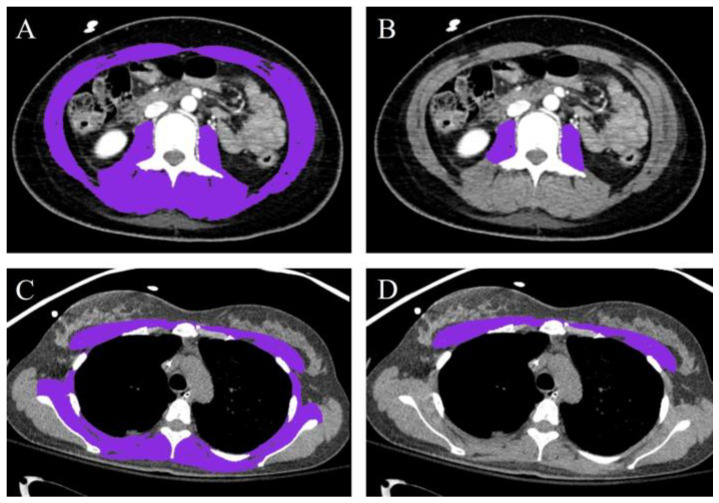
Cross-sectional CT images of the SMA indicated in purple. (A) L3 level SMA, (B) psoas muscle area, (C) T4 level SMA, and (D) pectoralis muscle area. Abbreviations: CT, computed tomography; L3, the third lumbar vertebra; SMA, skeletal muscle area; T4, the fourth thoracic vertebra.
